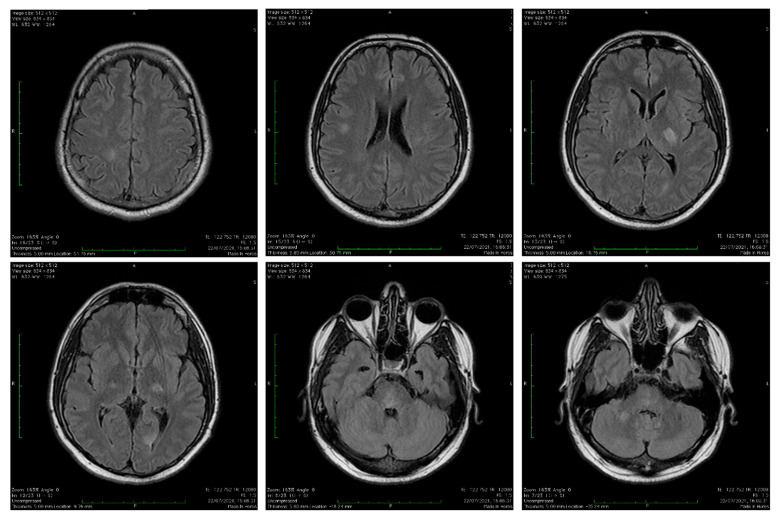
Axial cerebral MRI sequences. Multiple hyperintense lesions in the cerebral hemispheres, brainstem, and cerebellum.
